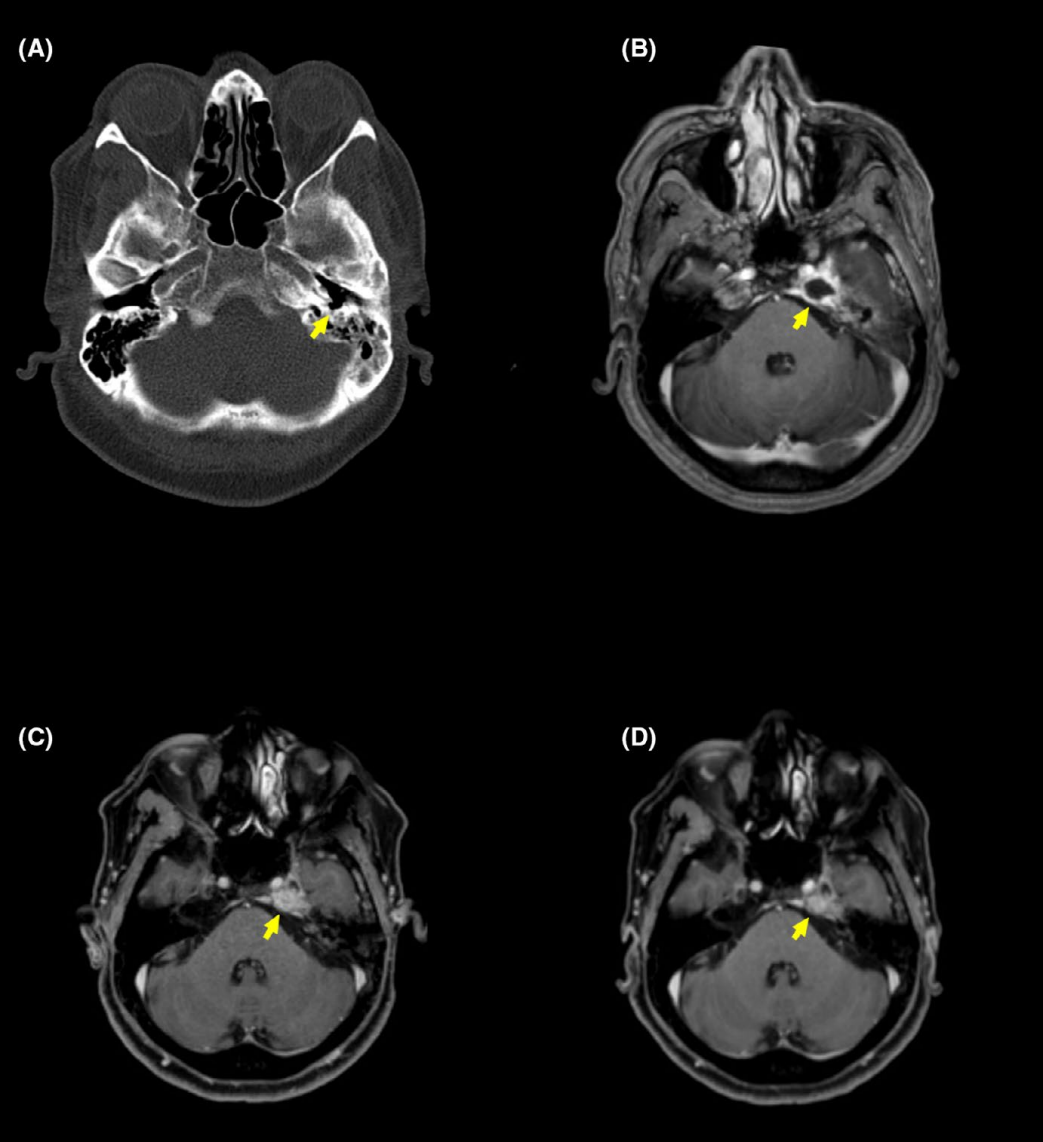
Imaging scans performed during patient's evaluation. A, Brain HR‐CT scan showing a bone gap (arrow) in the posterior segment of the external auditory canal. B, Brain MRI performed on day 8 pointing an inflammatory process in the left mastoid associated with an 2 cm abscess (arrow) in the left petrous apex extending to the dura mater. C, Brain MRI performed on the 33rd day pointing an improvement of the inflammatory process in the left mastoid‐associated abscess size reduction (arrow). D, Brain MRI performed 52 d after the first brain HR‐CT scan. Brain MRI was performed 8 d after discharge to evaluate response to treatment, showing no evidence of central sinus thrombosis, complete abscess resolution (arrow), absence of the signs of insinuation to the left pontocerebellar cistern, and partial obliteration of Meckel's cave
